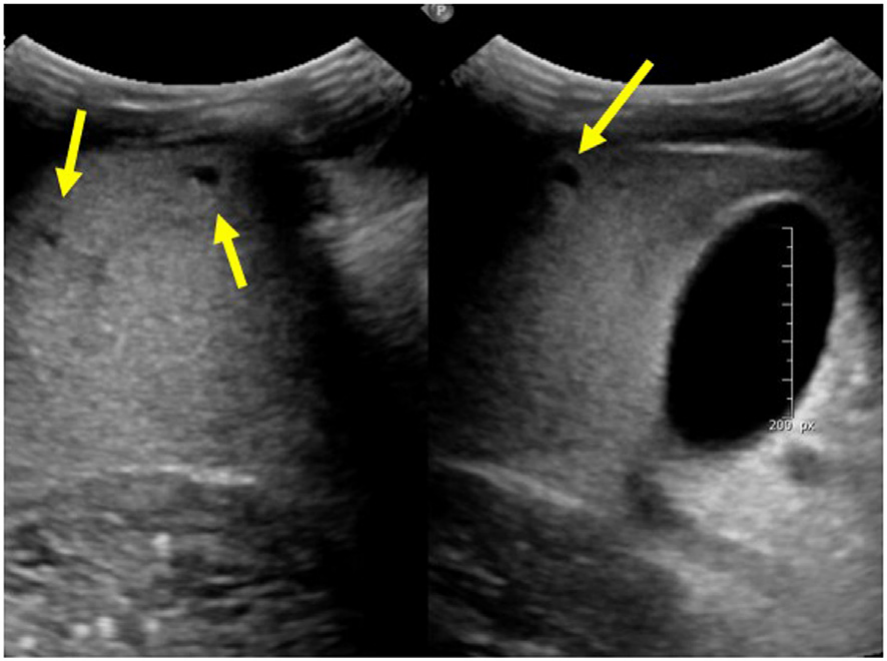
Ultrasound images of the liver showing multiple hepatic cysts in a patient with autosomal recessive polycystic kidney disease.
